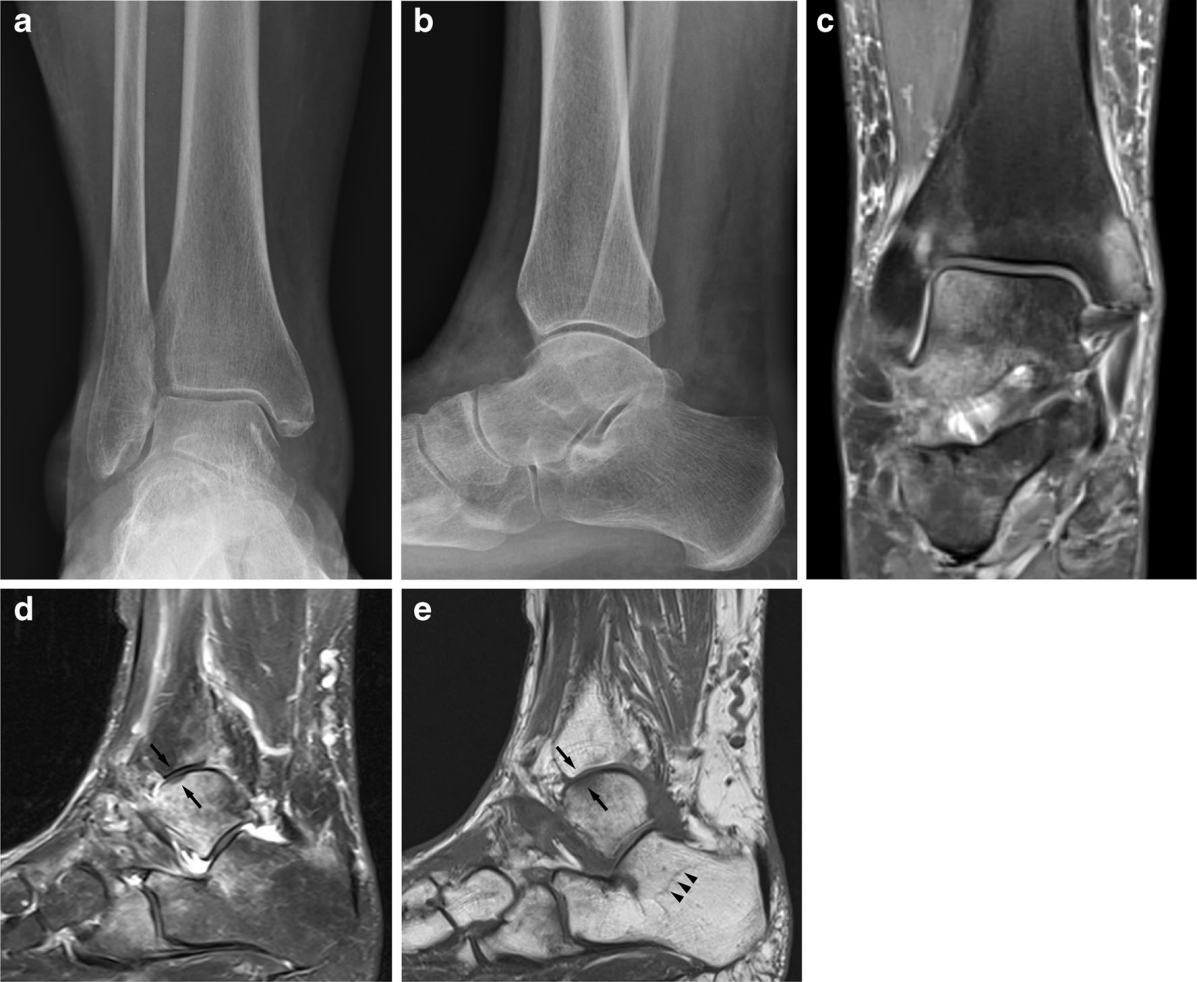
An elderly female patient with foot pain. AP (a) and lateral (b) radiographs of the ankle show osteopenia but do not show a reason for significant foot pain. Coronal (c) and sagittal (d) PD-FS sequences show significant bone marrow oedema-like changes in the medial malleolus, lateral ankle joint, calcaneus and navicular and cuboid. In the lateral talar trochlea there is focal low signal change in fluid sensitive (d) and T1w sequences (e) consistent with focal subchondral infraction (black arrows). The sagittal T1w sequences (e) demonstrate low signal lines in the cancellous bone indicating stress fracture lines in cancellous bone (arrowheads)
